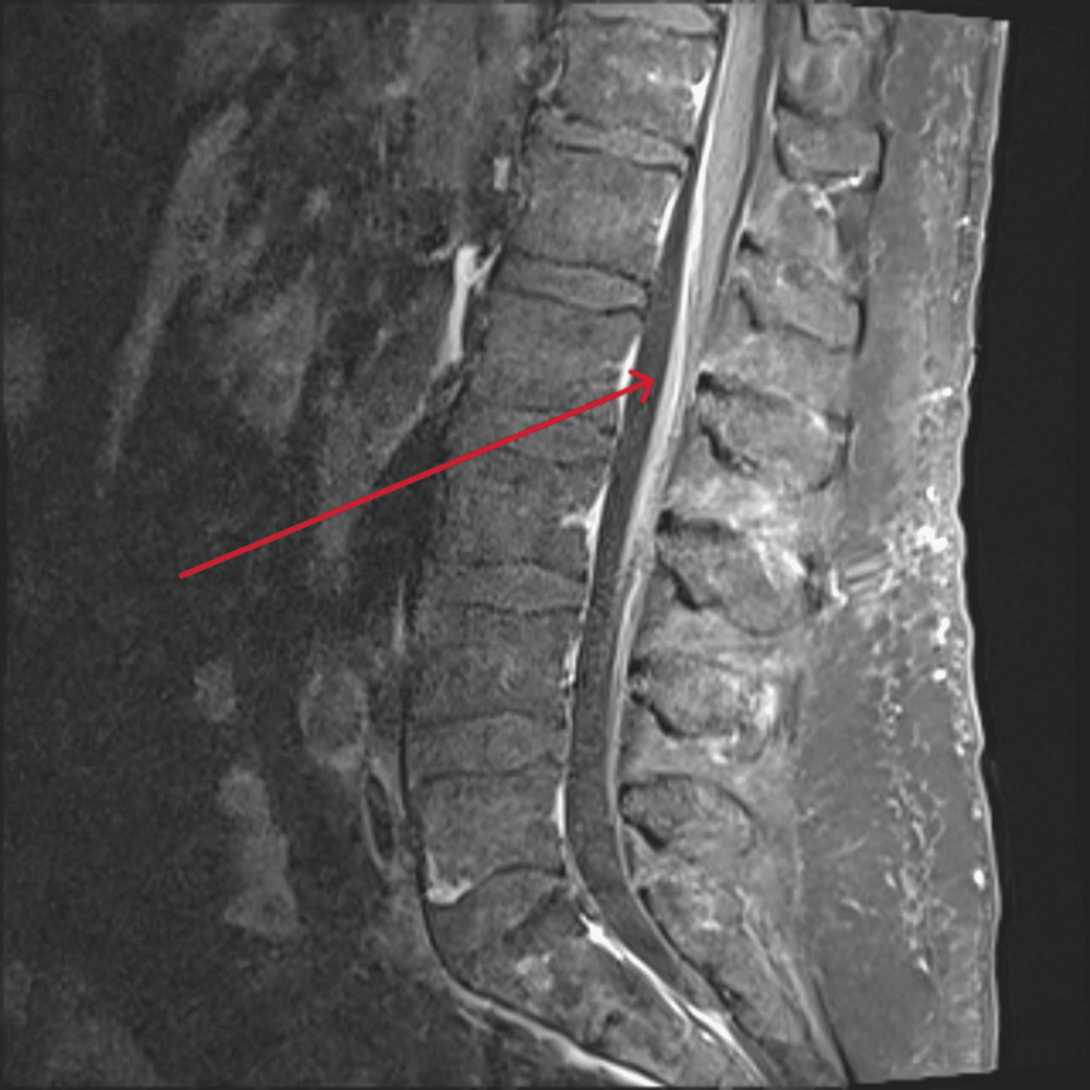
MRI showing abnormal enhancement of ventral rootlets in the lumbar spine MRI: Magnetic resonance imaging
